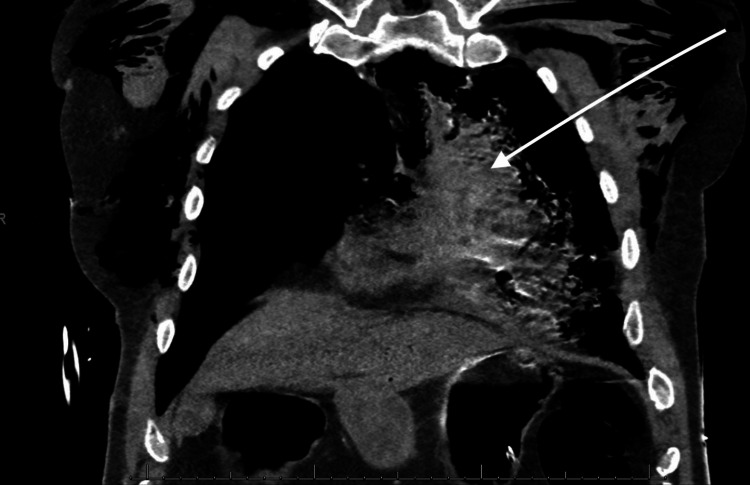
CT scan of the chest coronal view showing hemopericardium (see white arrow)
